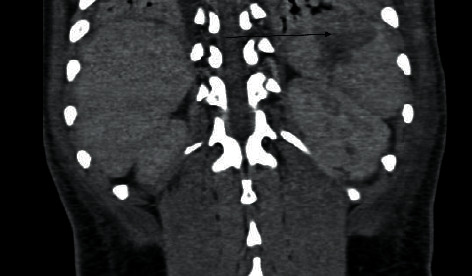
Computed tomography (CT) of the abdomen showing hypodense pyramidal wedge-shaped defect consistent with splenic infarcts (black arrow).
